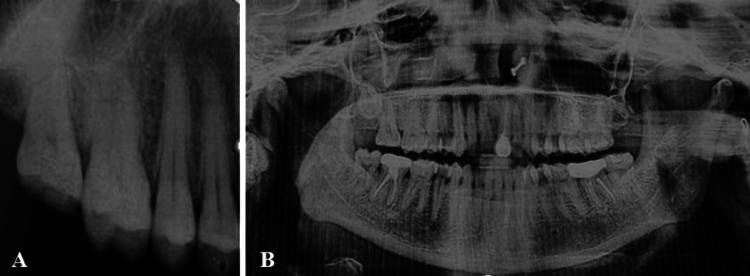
Radiographic Findings (A) Intraoral periapical radiograph showing no local osseous abnormality, except for an impacted right maxillary third molar (tooth 18). (B) Orthopantomogram demonstrating an impacted right maxillary third molar (tooth 18).
